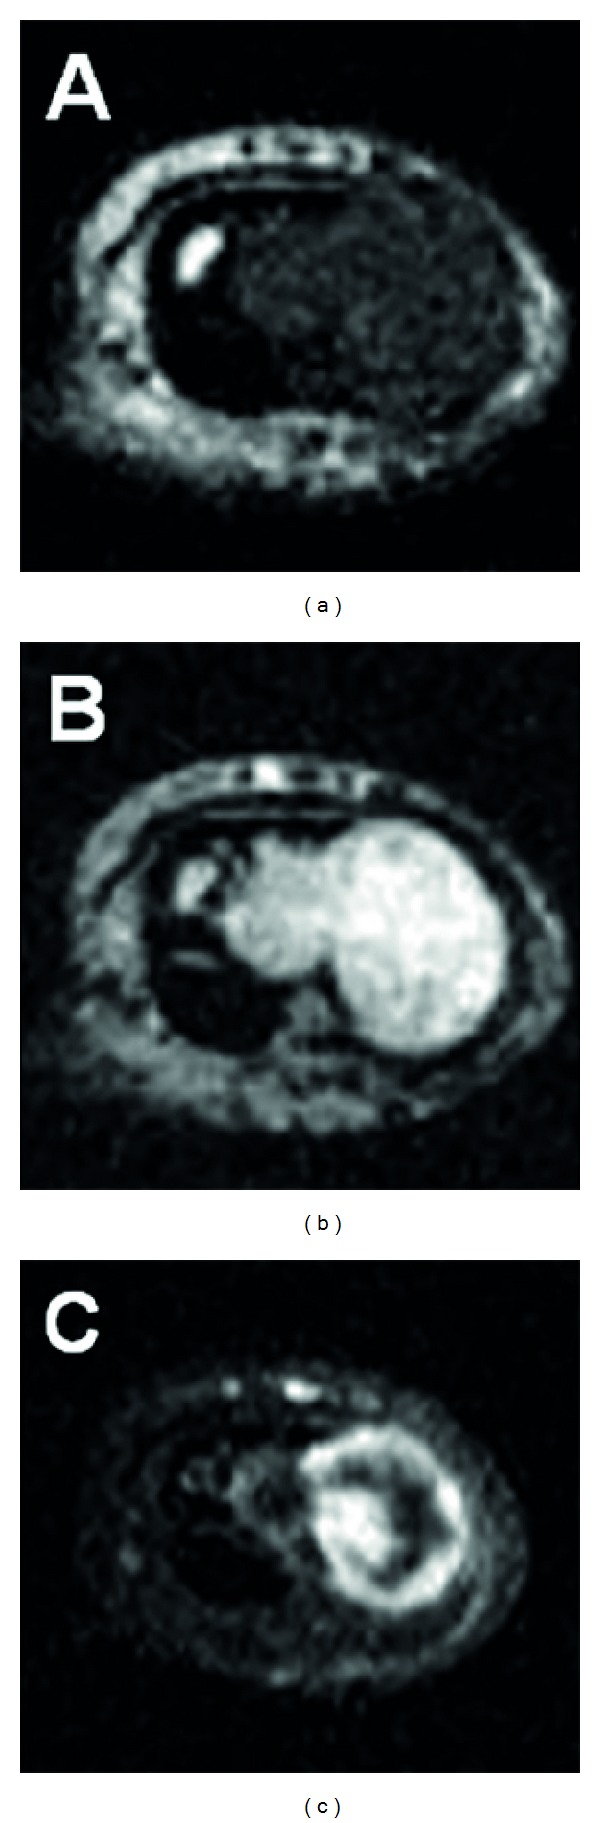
Axial magnetic resonance imaging showing a well-defined juxtacortical mass with intermediate signal intensity on T1-weighted image (a) and high signal intensity on T2-weighted image (b). Contrast-enhanced fat-suppressed T1-weighted image (c) demonstrates peripheral and septal enhancement.
